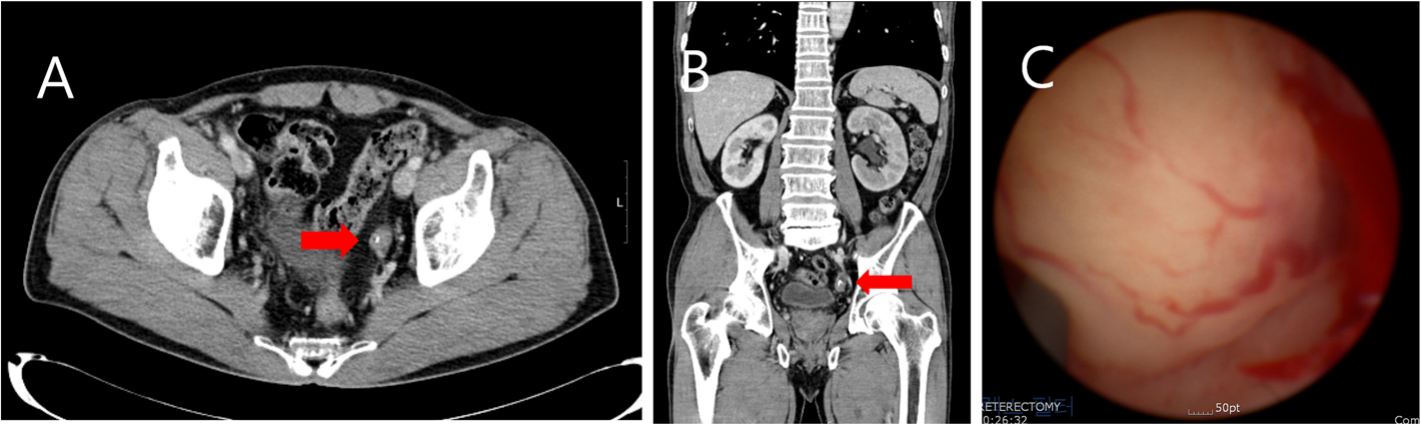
Radiography and ureterorenoscopy. (A,B). Abdominal computed tomogram showing a stone in the left distal ureter and a mildly enhanced fungating mass lesion just below the stone-impacted site (red arrow). (C) Ureterorenoscopic view showing a huge, round, fungating mass with a smooth surface filling the lumen of the left distal ureter.
